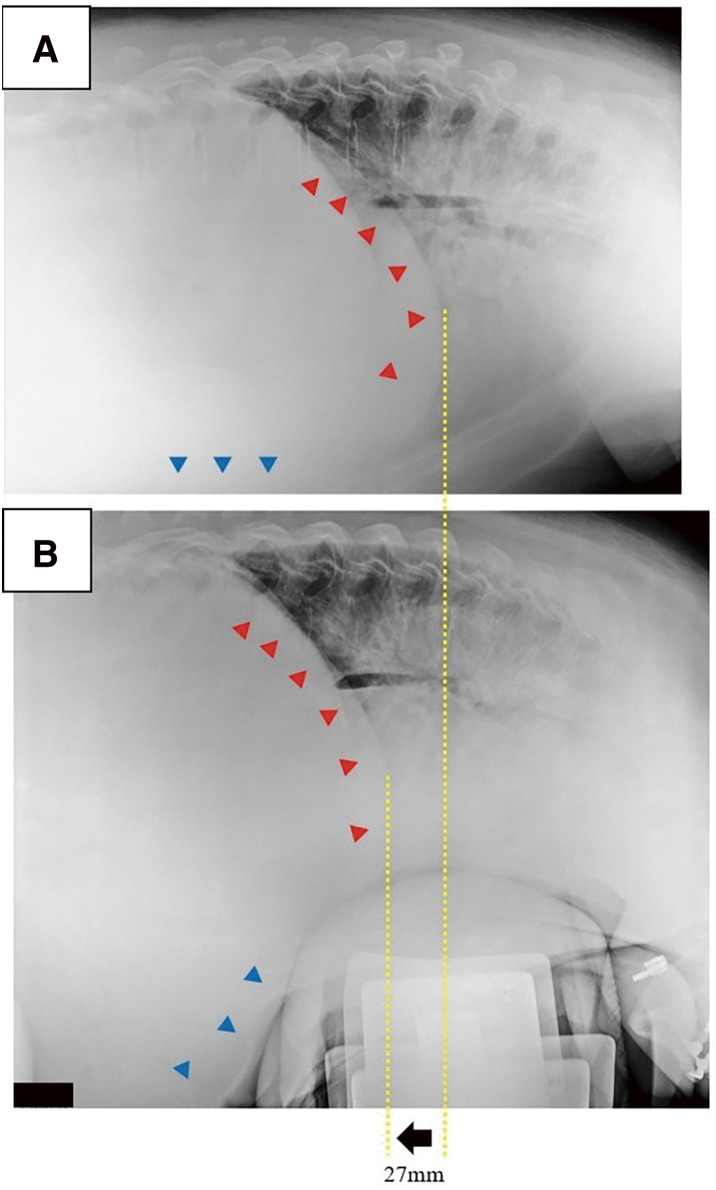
Lateral chest radiographs obtained at end-expiration in a patient with a BMI of 37 kg/m^2^ (A, B) showed a 27-mm caudal displacement of the diaphragm with the 4-point support frame compared with a mattress (red arrowheads, yellow dotted line), without change in the anteroposterior thoracic diameter, indicating reduced abdominal compression by allowing the abdomen to hang freely (blue arrowheads). BMI, body mass index
